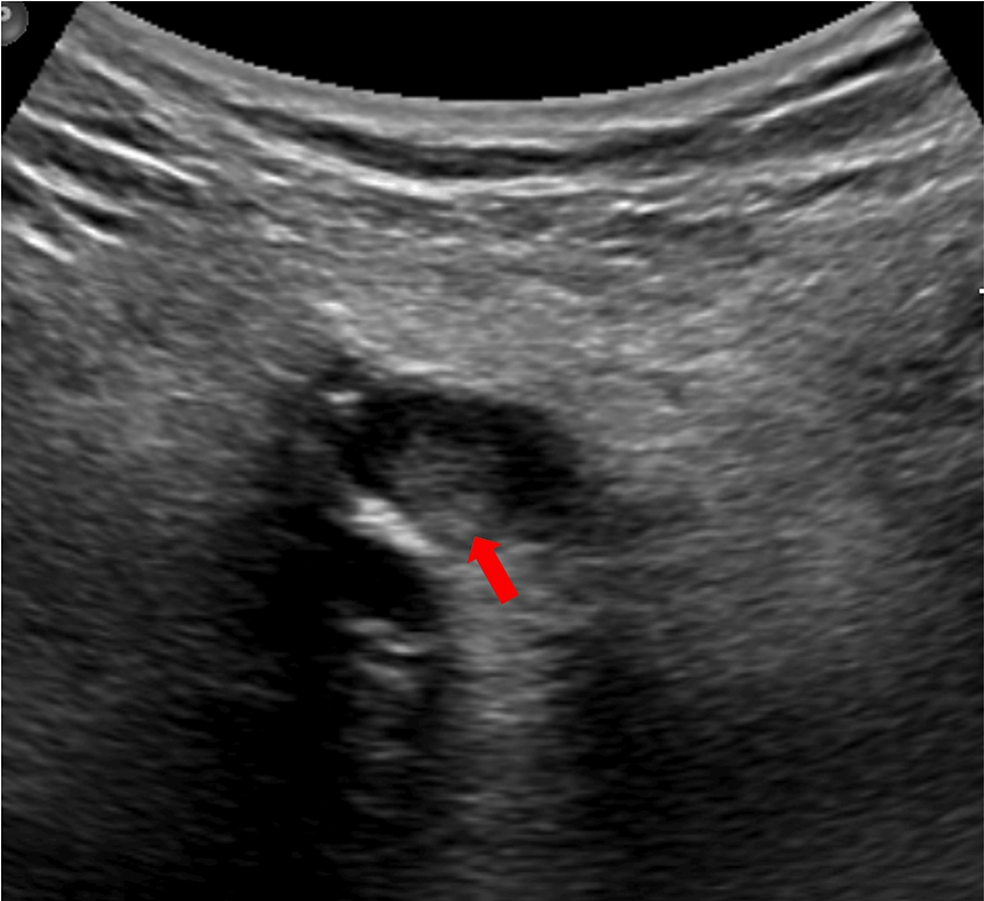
Ultrasound of the left lower limb Compression ultrasound of the left lower limb showing echogenic material (red arrow) and an absence of collapse upon compression in the popliteal vein
